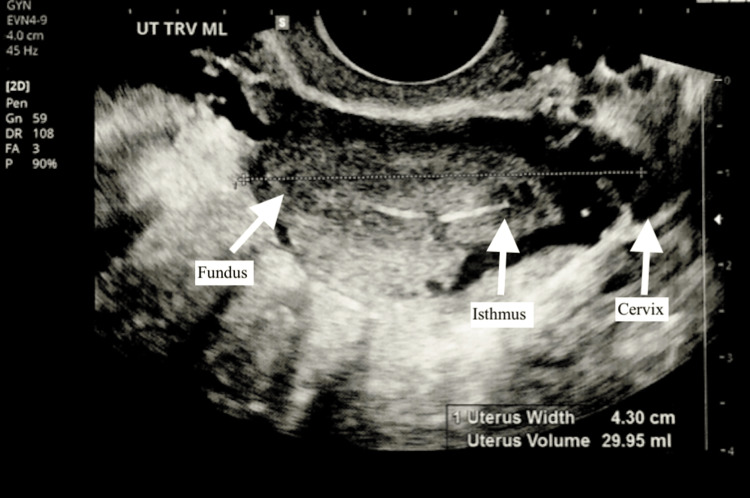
Transvaginal ultrasound of midsagittal view of uterus with arrows labeling fundus, isthmus, and cervix
